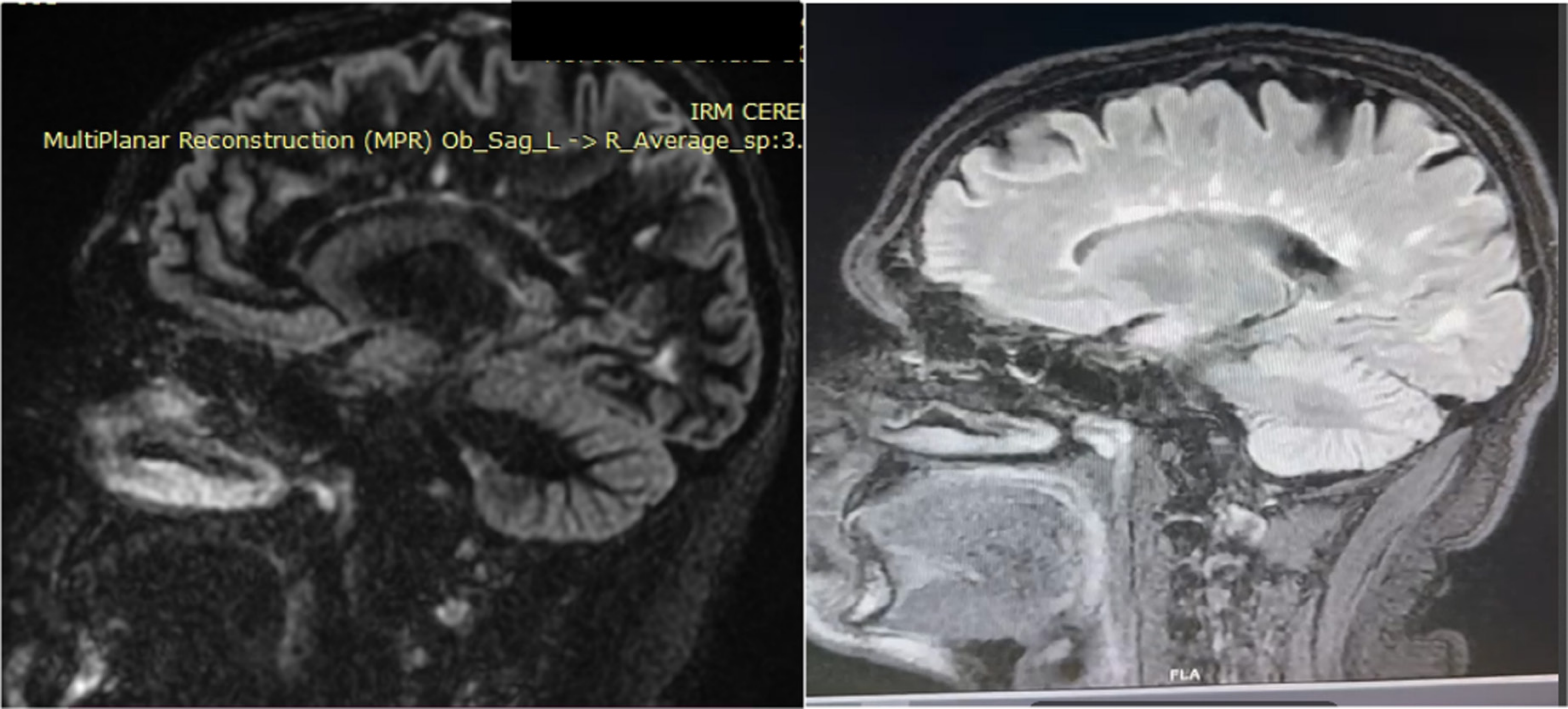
MRI year X+1 showing Dawson's finger sign.
